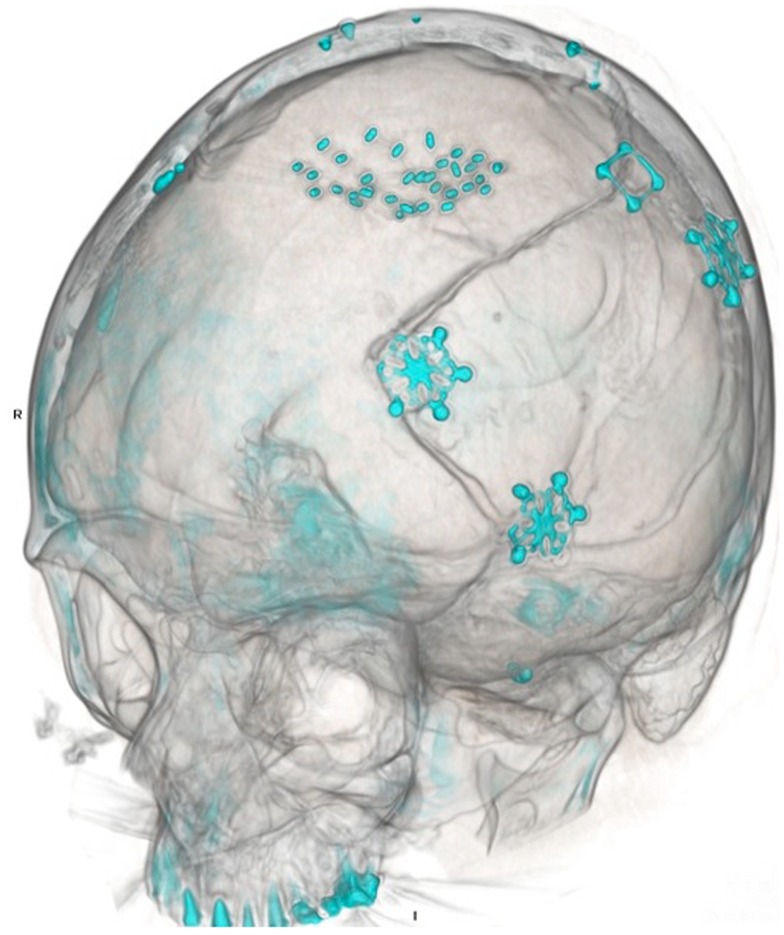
Post-operative CT scan. Three-dimensional reconstruction demonstrates both prior craniotomies with microplate fixation, as well as radioactive brachytherapy seeds placed in the resection cavity.
